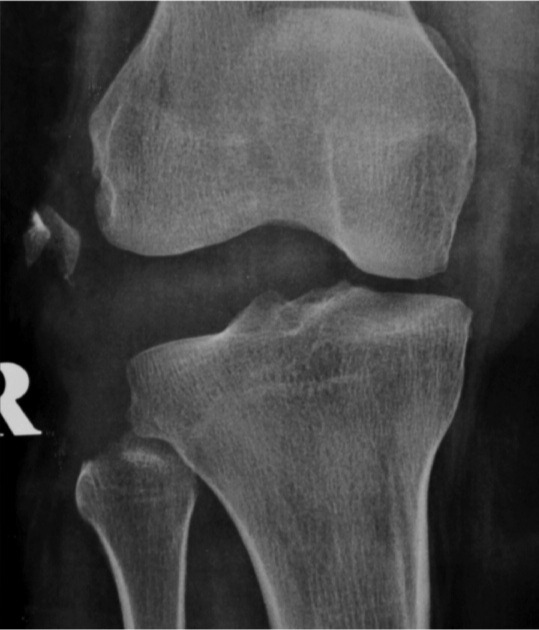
Initial radiograph right knee showing right knee dislocation.
